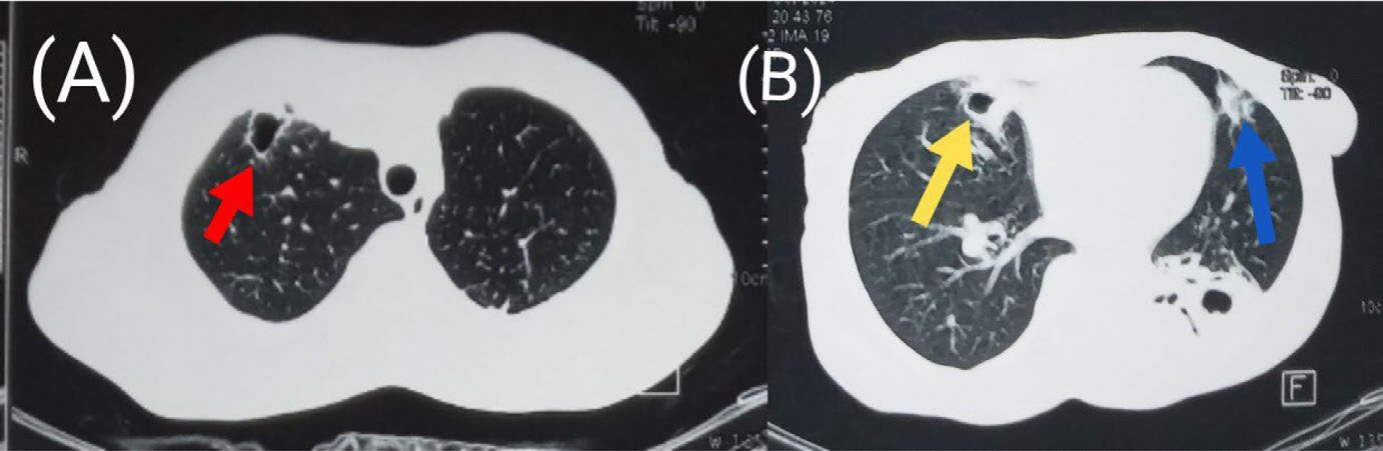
Pre‐contrast axial CT sections of the chest in lung window showing cavities with resolved air‐fluid levels in the right upper (red arrow in A) and middle (yellow arrow in B) lobes. Also noted is nearly complete resolution of the smaller cavity in the superior lingular segment of the left upper lobe (blue arrow in B).
